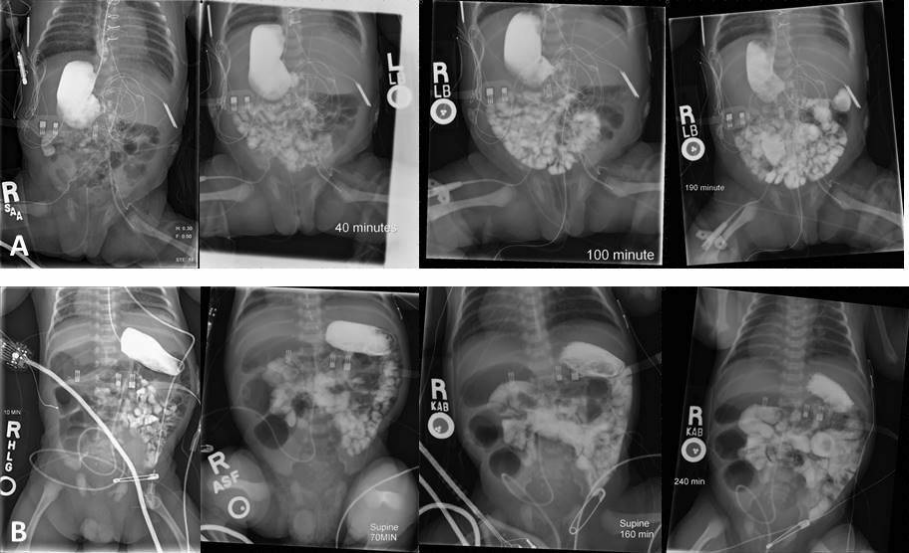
Intestinal malrotation Abdominal radiographs from upper gastrointestinal series from two separate patients with malrotation without obstruction.
